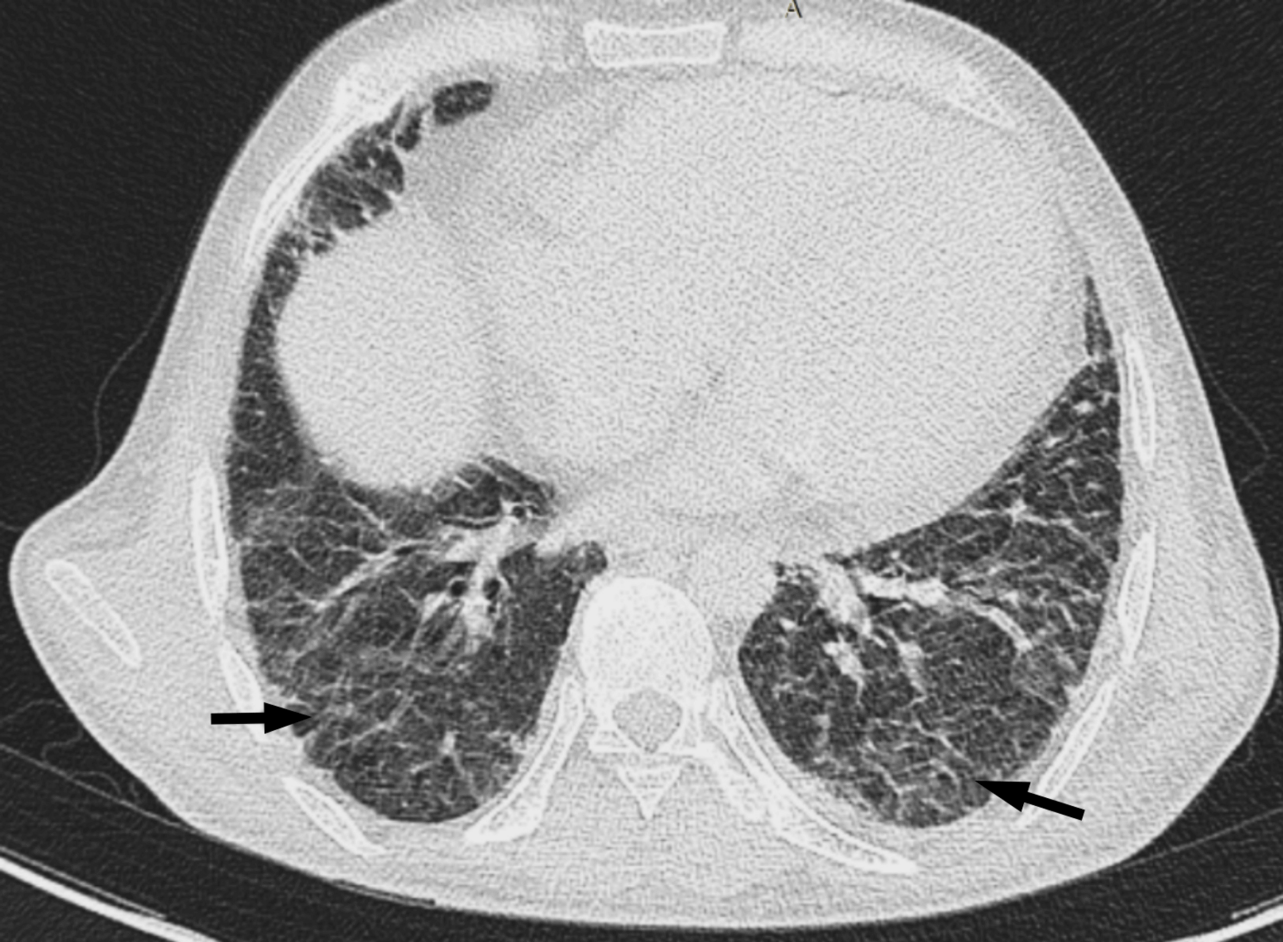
CT chest Bilateral subpleural reticular opacities (arrows).
